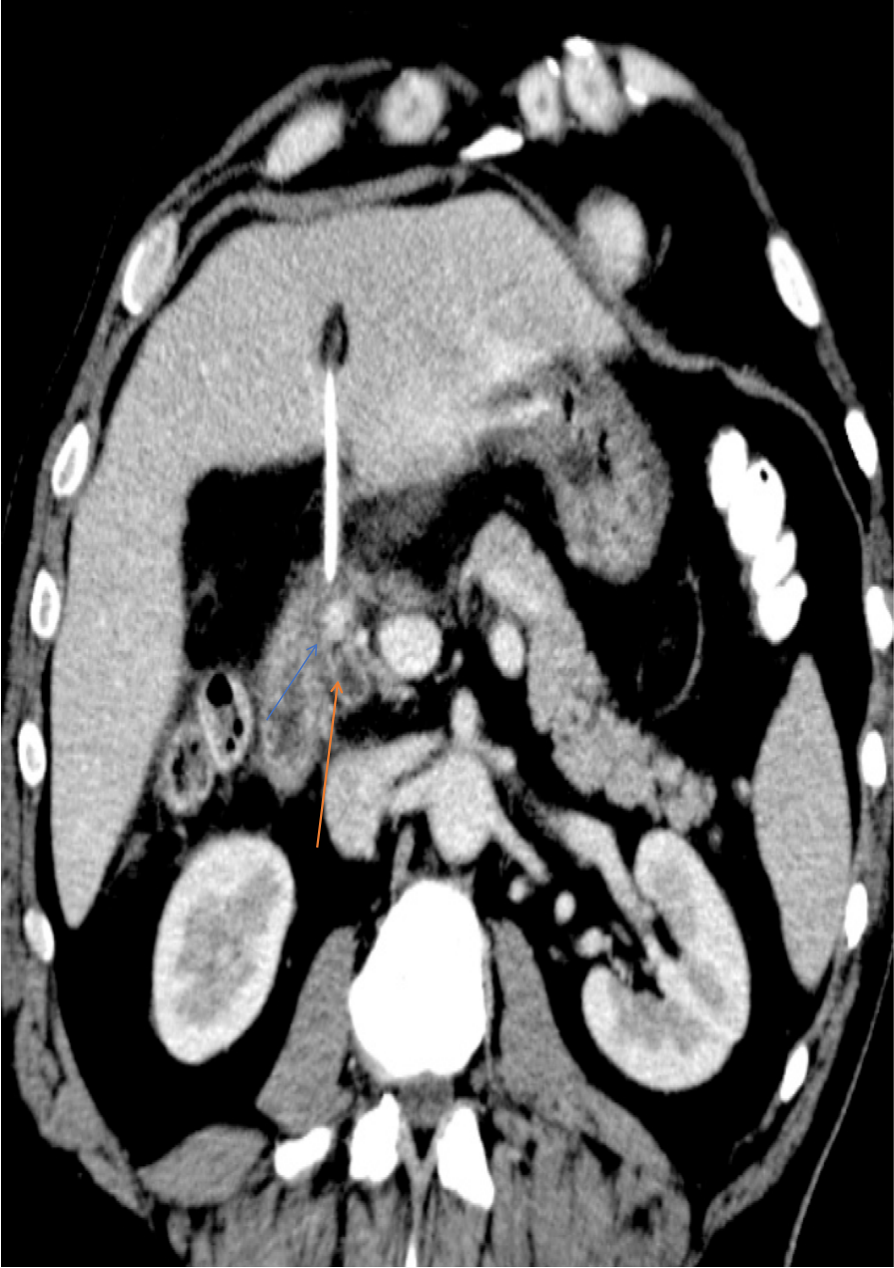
CT-angiography showing a pseudo-aneurysm of the gastroduodenal artery with probable erosion of the main bile duct
